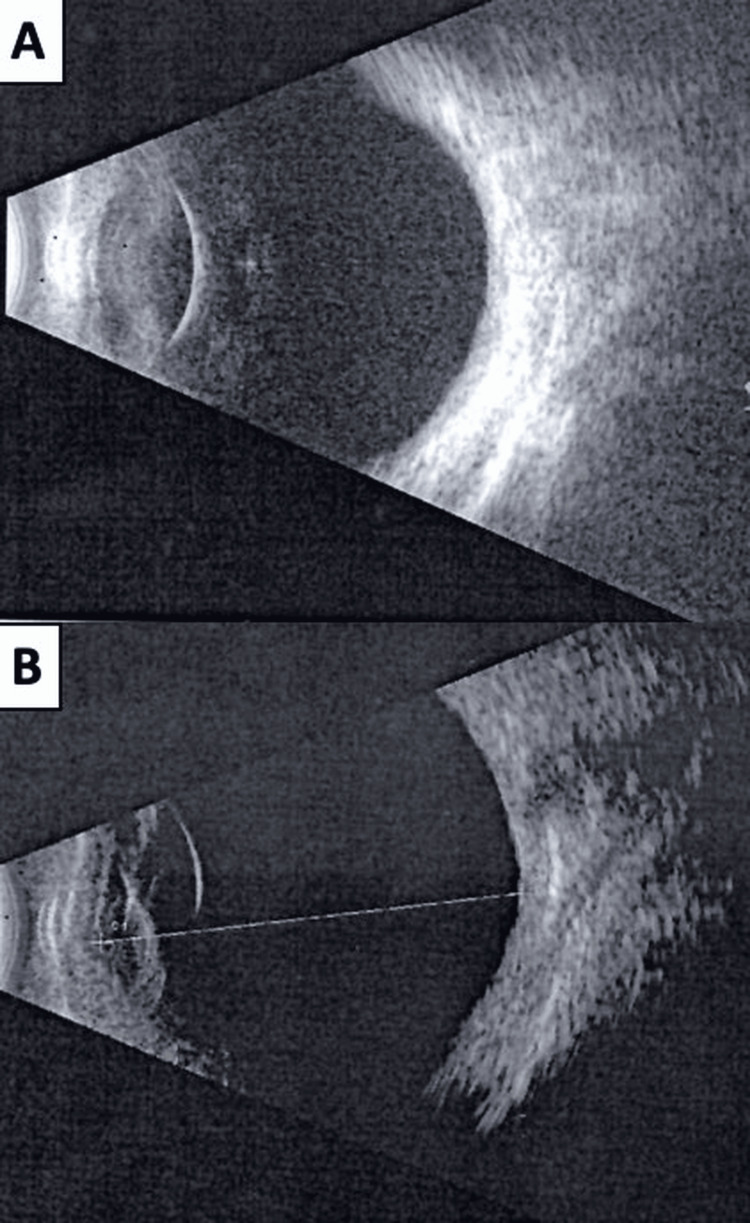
Ocular ultrasound (B-scan) showing a normal posterior segment: (A) right eye and (B) left eye
